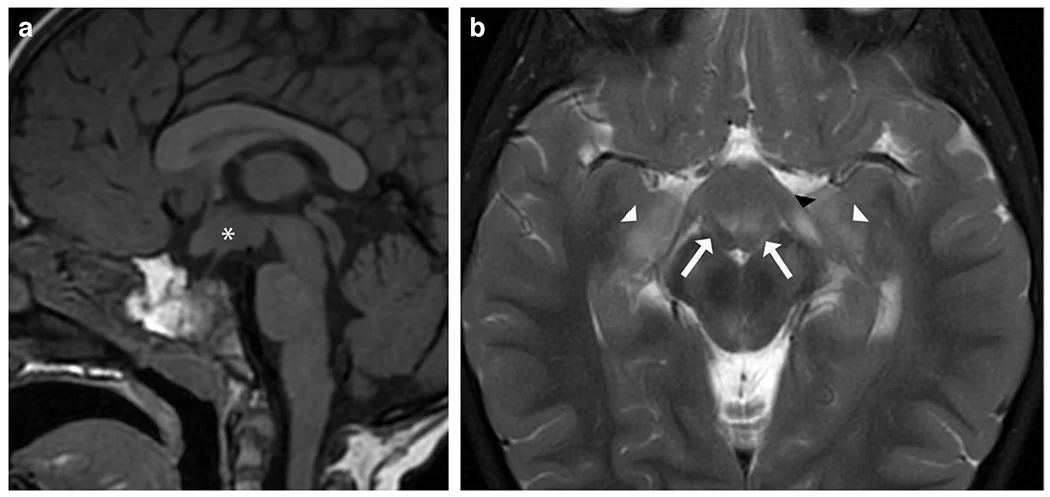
Optic pathway glioma in a 3-year-old female with a known history of neurofibromatosis type 1 presenting with growth hormone excess. a Sagittal T1-weighted image through the midline shows a T1-isointense 2-cm mass (*asterisk*) centered at the optic chiasm. b Axial T2-weighted image shows the lesion (*arrows*) centered at the level of the hypothalamus between the optic tracts and the cerebral peduncles. Hyperintense T2-weighted signal is partially visualized in the left optic tract (*black arrowhead*), consistent with involvement by optic pathway tumor, and bilateral hippocampal formations (*white arrowheads*), reflecting the myelin vacuolar changes typical of neurofibromatosis type 1
